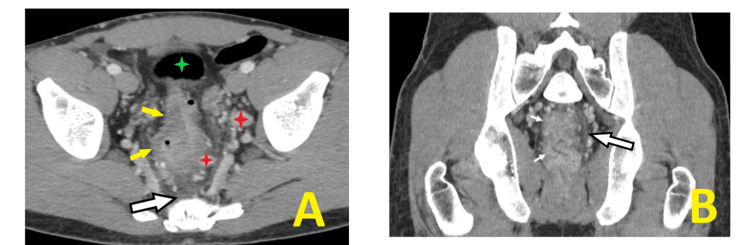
Contrast-enhanced pelvic CT demonstrating inflammatory proctitis A. Contrast-enhanced axial CT of the pelvis shows diffuse rectal wall thickening and submucosal edema (small yellow arrows), perirectal and presacral inflammatory fat stranding (larger white arrow with black border), and prominent mesorectal and left pelvic sidewall lymphadenopathy (red stars). Note the transition to a normal upstream bowel wall in the sigmoid colon (green star). B. Contrast-enhanced coronal CT of the pelvis shows similar findings of diffuse rectal wall thickening (small white arrows) and mesorectal inflammatory fat stranding (larger white arrow with black border).
